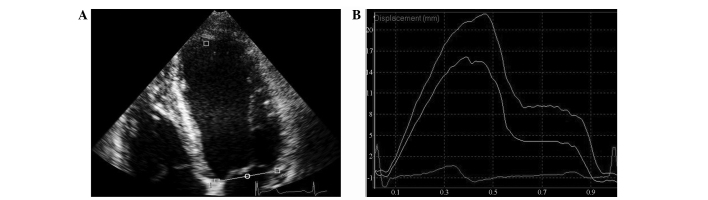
Apical four-chamber view for MAD measurement by the TMAD method and a displacement-time curve. (A) The software was started, three points were selected (lateral interventricular septum of mitral ring, lateral wall side of mitral ring and the apex), and the mitral annulus sampling points were marked. (B) Left ventricular lateral wall and posterior septum displacement-time curves. The displacement-time curves corresponded with the sampling points (in color, not shown), which enabled them to be quickly read. MAD, mitral annular displacement; TMAD, tissue motion annular displacement.
